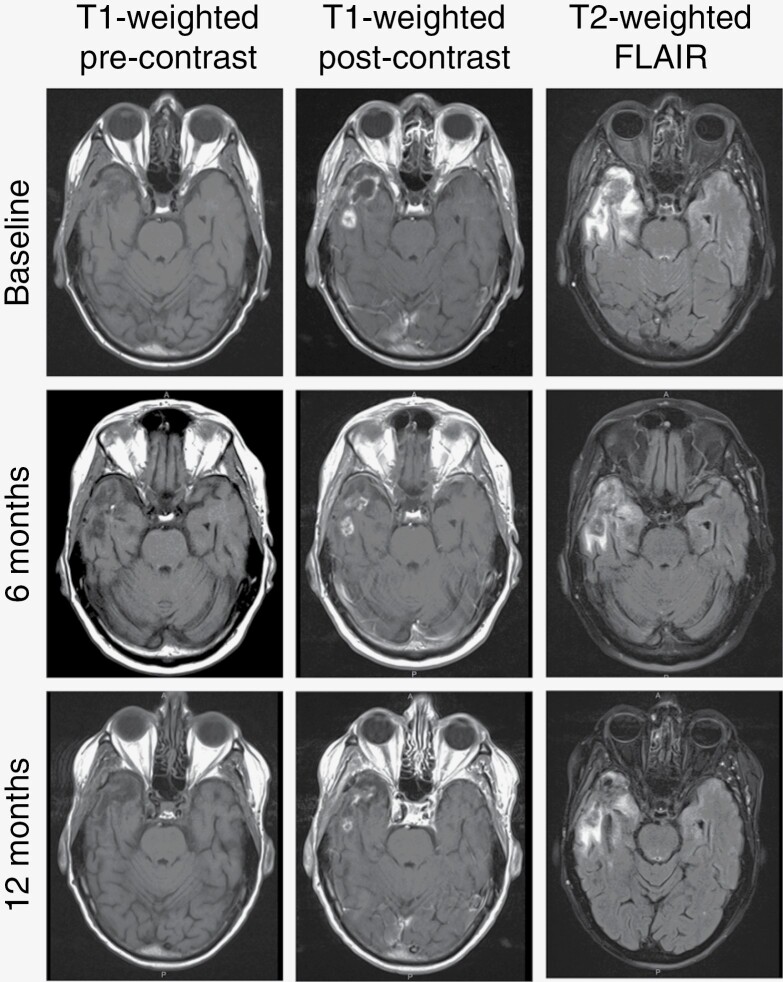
Radiographic demonstration of treatment response. Representative axial MRI sequences demonstrating partial treatment response at 6 and 12 months in a 68-year-old patient with a right temporal GBM, *IDH*-wildtype tumor. Baseline scan obtained approximately 4 months following diagnosis and after concomitant TMZ/RT.
